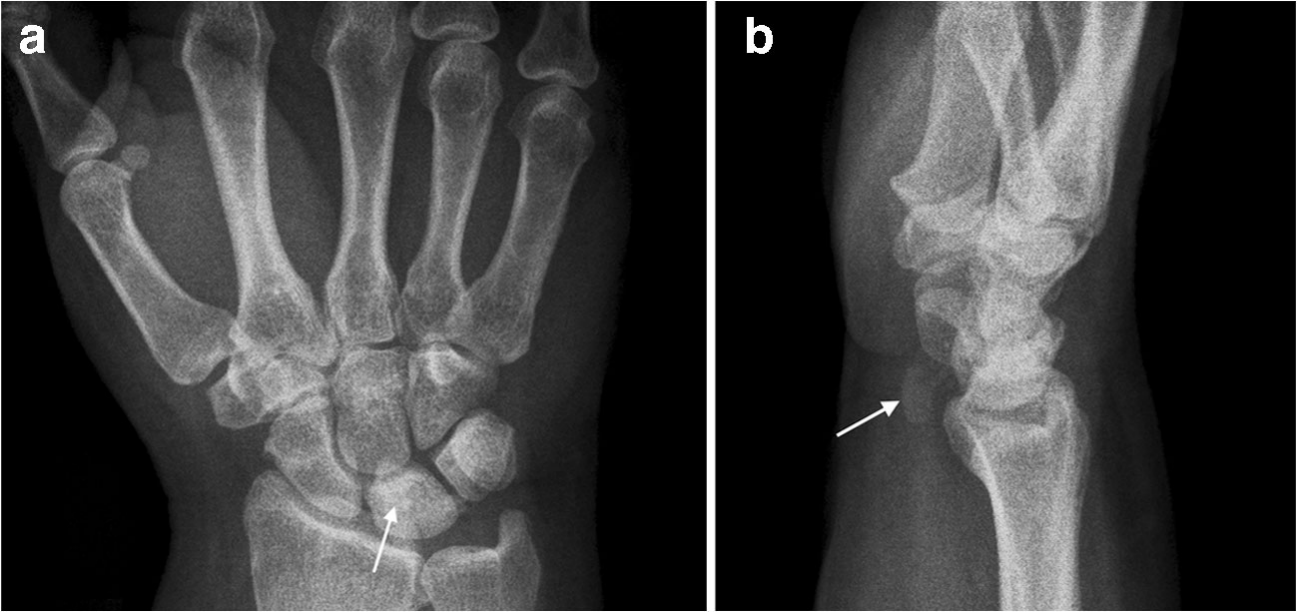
Radiographs demonstrating a solitary amorphous calcification at the volar site of the right wrist adjacent to the proximal carpal row (b, arrow). There are no signs of erosions or carpal malalignment. Note the slightly sclerotic lunate bone (a, arrow)
